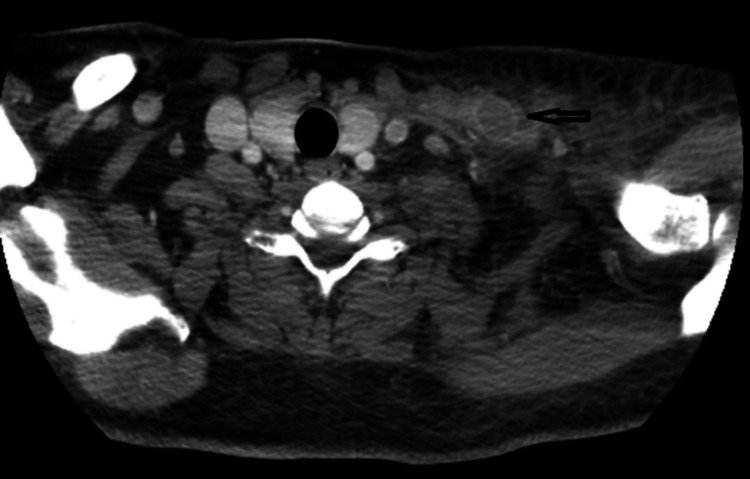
CT scan of neck axial view showing thrombus in the left external jugular vein (black arrow)
